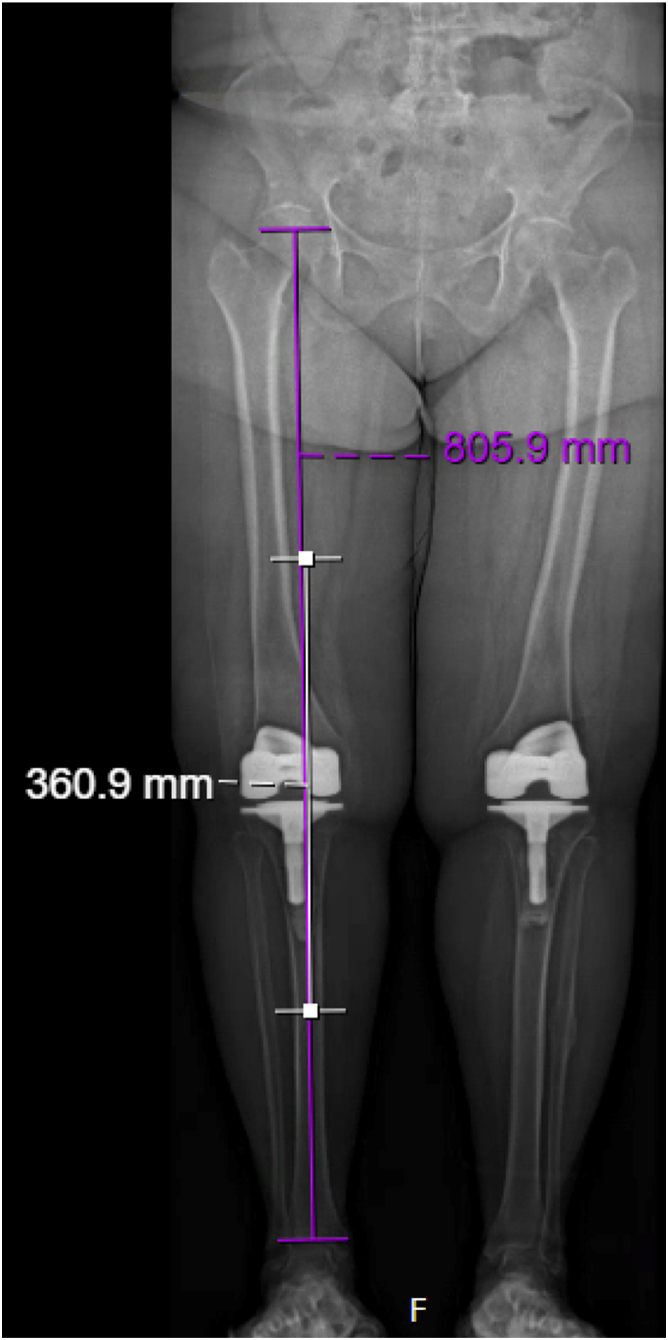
The length of the femoral nail is selected based on preoperative long length radiograph to engage both the tibial and femoral diaphyses, measured here as a 360-mm nail.
